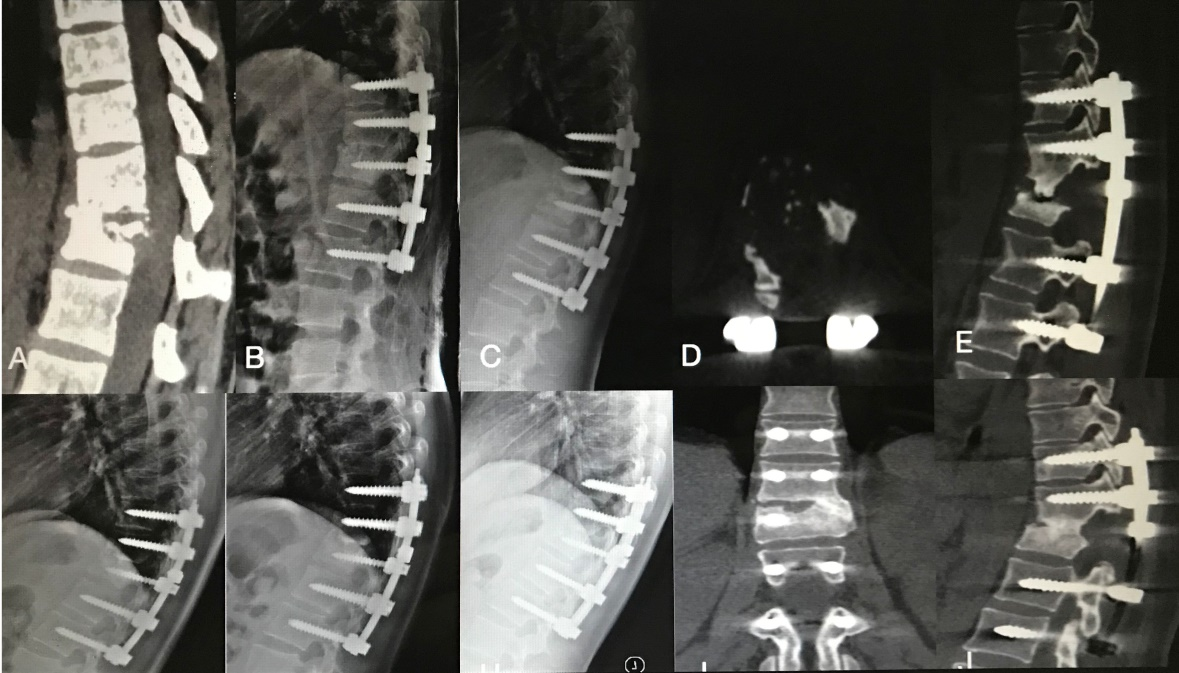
An 22-year-old female patient with thoracic spinal tuberculosis (T11-12) underwent posterior debridement and decompression combined with instrumentation. (A) Preoperative computed tomography (CT) showing bone destruction of the T11-12 vertebrae and compression of the spinal cord. (B,C,D,E) 1-week and 6-month postoperative X-rays and 6-month postoperative CT showing maintained correction and the bone wasn't fully healing. (F,G) 1-year and 2-year postoperative X-rays showed kyphosis correction have loss. (H,I,J)At 4.5-year follow-up, plain X-ray, and CT show solid bone fusion.
